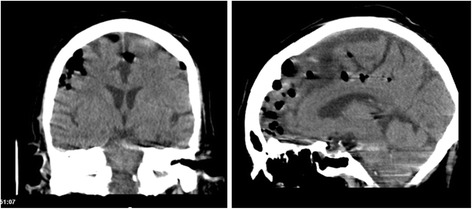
Coronal (left) and sagittal (right) CT imaging of cerebral hemispheres demonstrating multiple loculi of intracranial air
